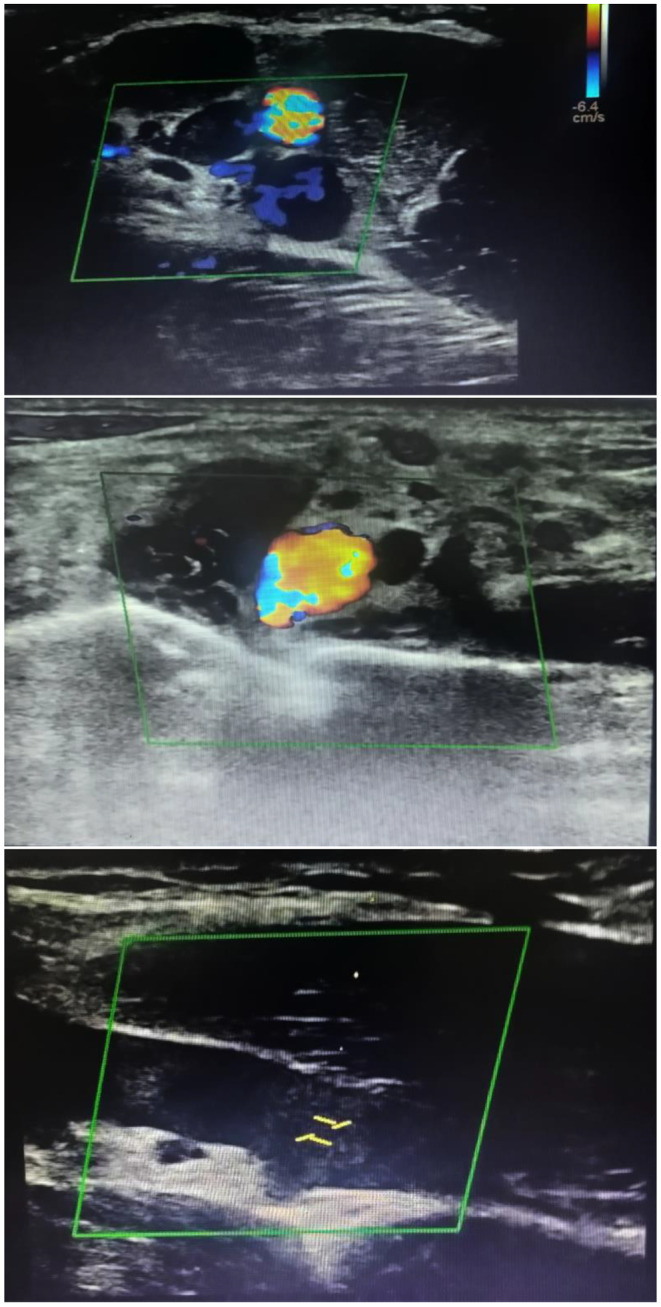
Venous duplex ultrasound of the right upper arm of our patient. The Doppler and B‐mode with absence of compressibility in the right basilic, axillary, and subclavian veins indicating a thrombosis of the studied veins. The pulsed Doppler spectral waveform exhibits an absence of flow augmentation indicating a venous obstruction at the level of the right basilic vein.
